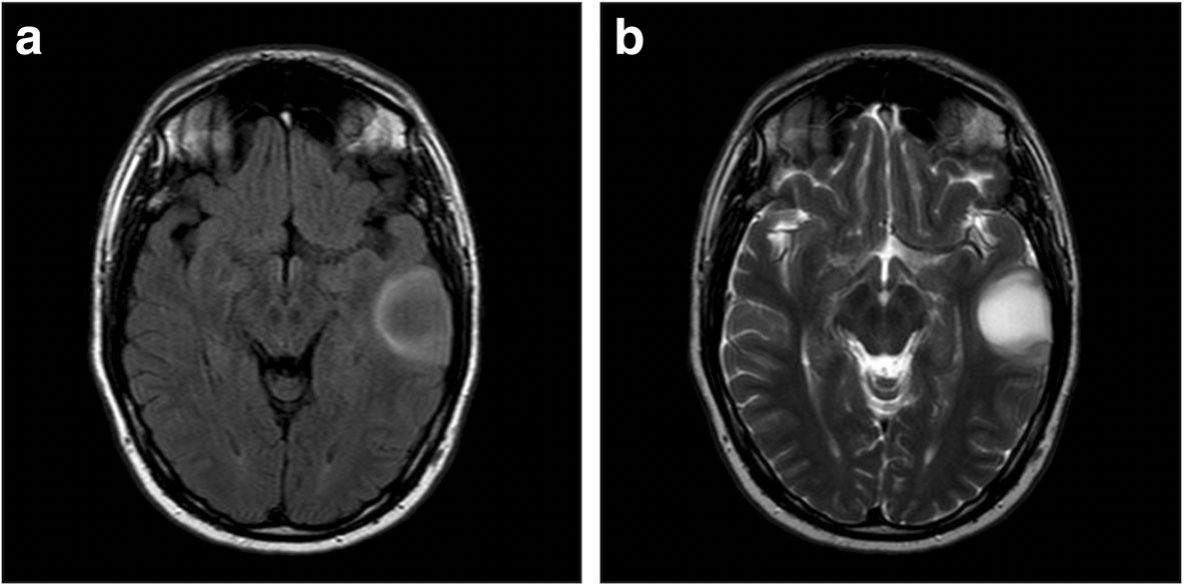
a-b: a) FLAIR sequence demonstrating a relative hypointense signal with the exception of a hyperintense peripheral rim. b) T2W sequence demonstrating homogenous hyperintensive signal with a conspicuous border. This glioma was considered to have a mismatch sign and was diagnosed with an *IDH*-mutated and 1p19q codeleted glioma (i.e. oligodendroglioma)
